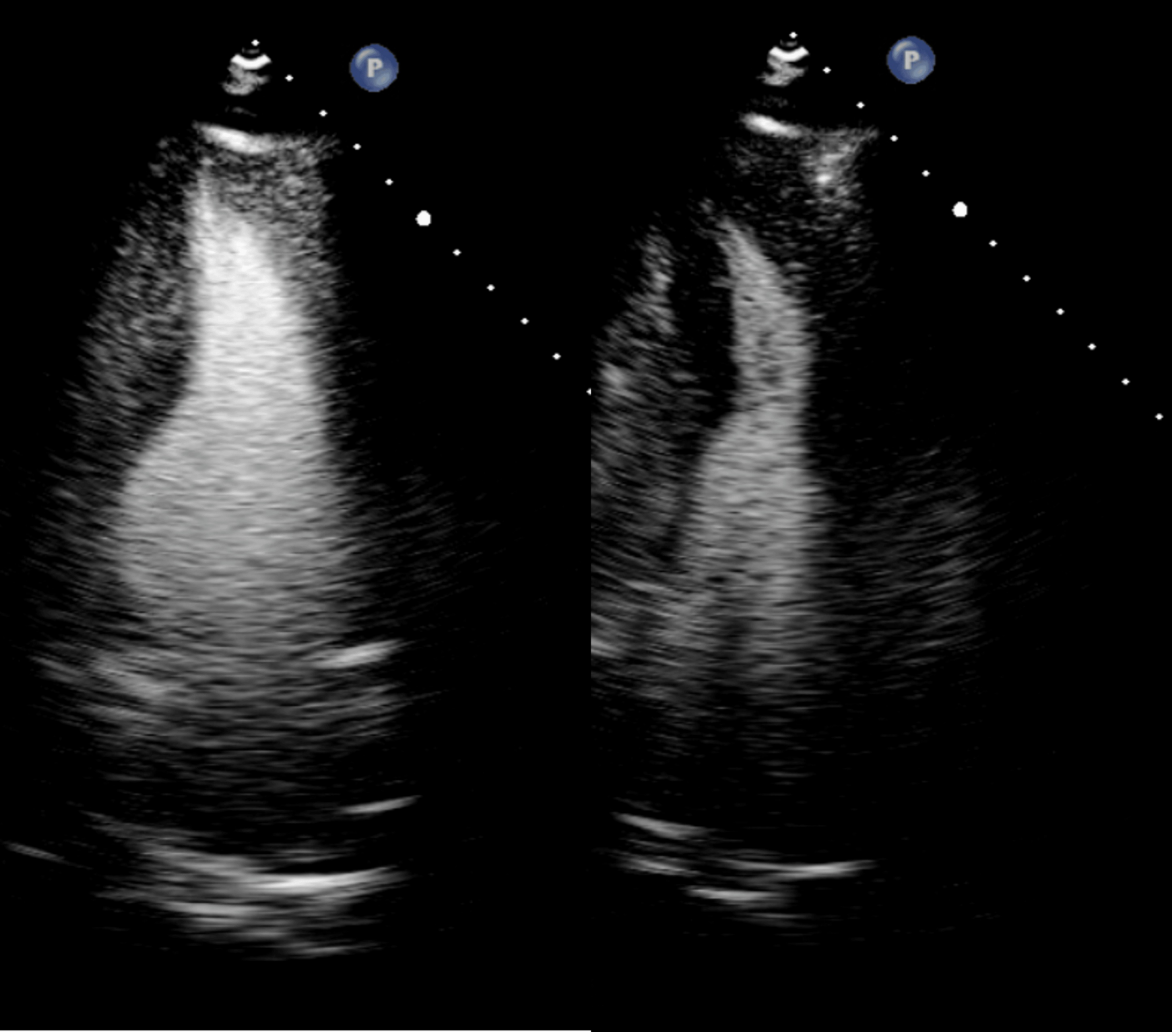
ApHCM TTE apical 2 chamber in diastole (a) and in systole (b) showing “spade-like” LV appearance. ApHCM: Apical hypertrophic cardiomyopathy; TTE: Transthoracic echocardiogram; LV: Left ventricle
